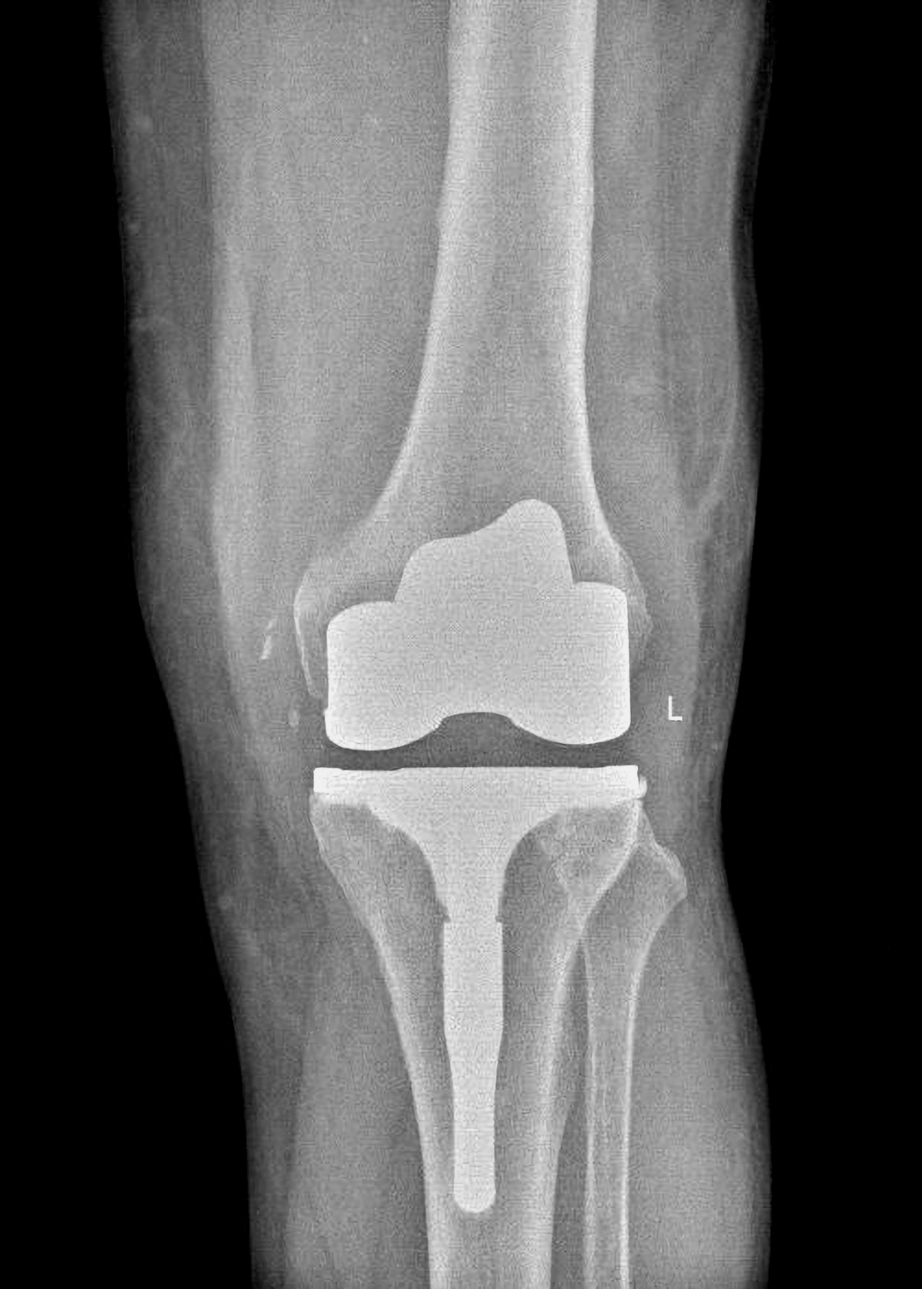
Anteroposterior X-ray of the Left Knee at Six Months
